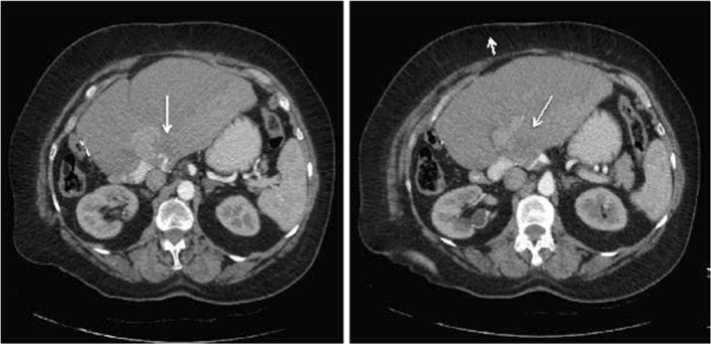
The CT scan 36 months after curative resection reveals intrahepatic tumor recurrence (*arrows*) arising from the left portal vein. The heterogenous texture of the liver on the right side of the tumor is caused by heterogenous perfusion of this hepatic area and is constant since primary surgery
